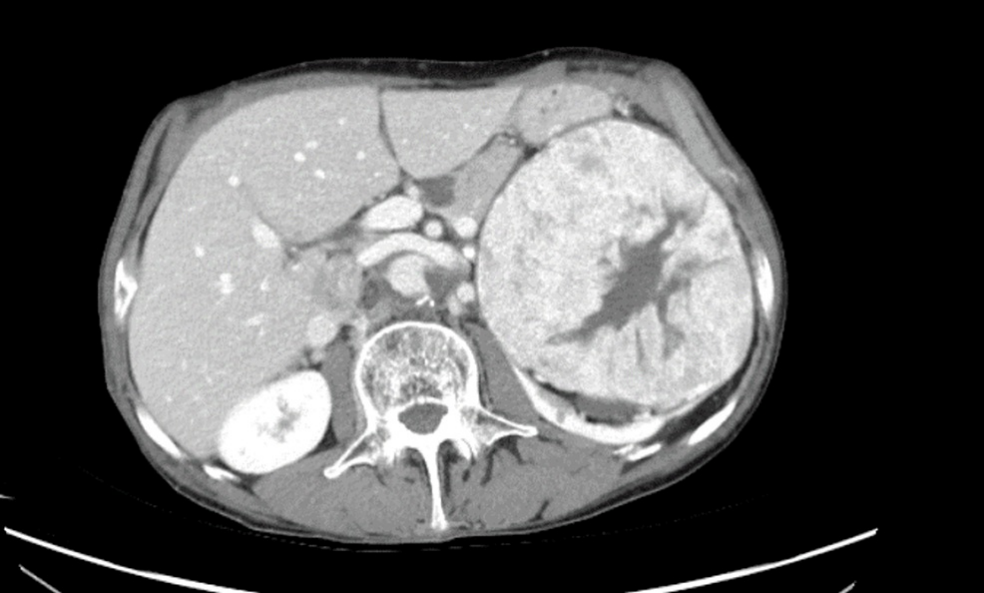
Axial image of CT angiogram showing renal mass with a central stellate scar.
